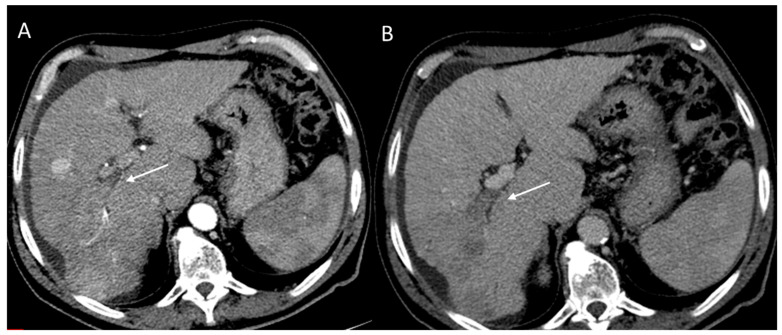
Tumor in vein. Portal thrombosis CT assessment during arterial (A) and portal (B) phase of contrast study.
